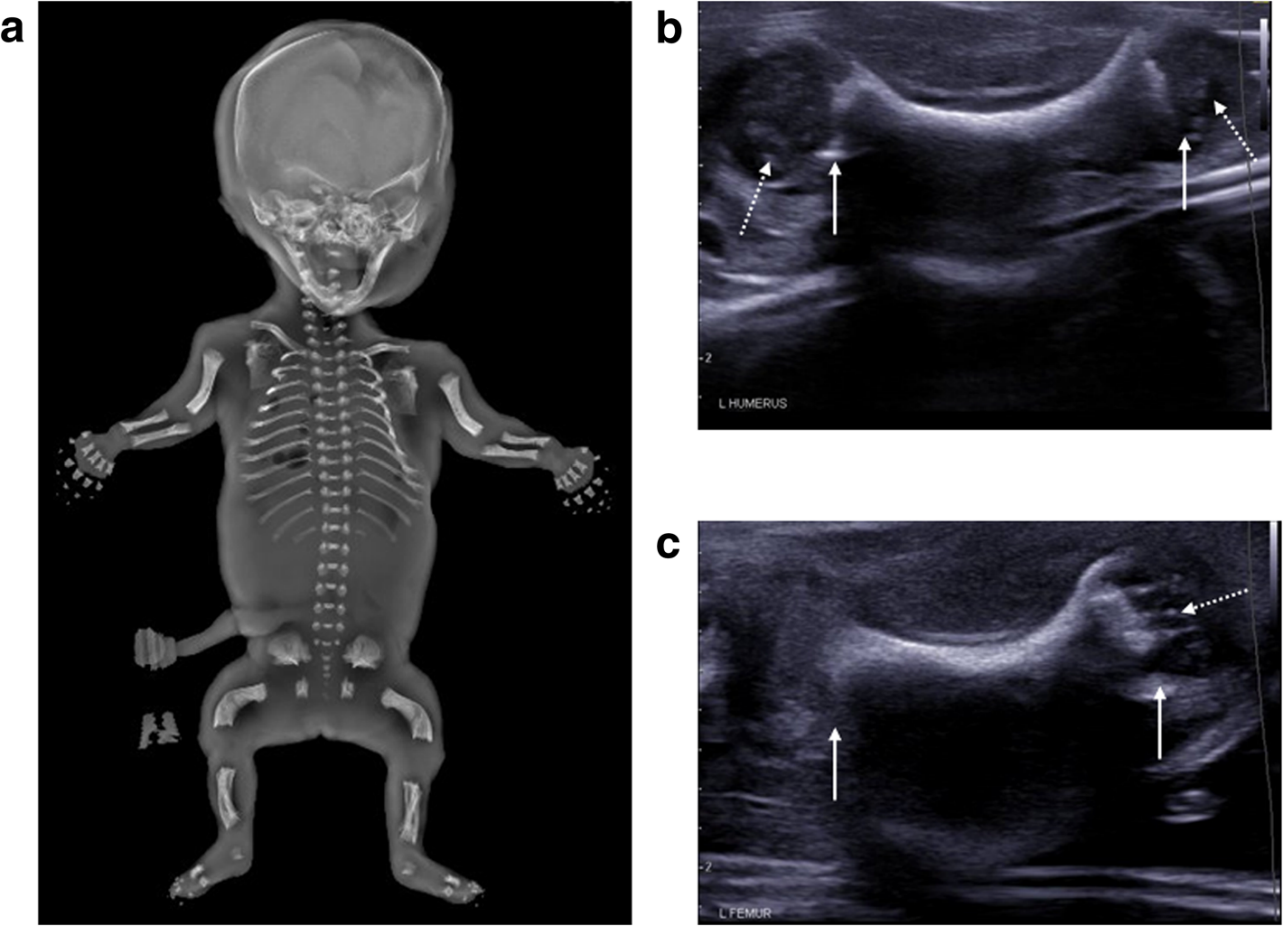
Thanatophoric dysplasia in a foetus at 22 weeks gestation, following termination of pregnancy. The frontal projection of the skeletal survey (a), acquired 2 days after delivery, demonstrates shortened long bones and curved femora with a trident appearance to the acetabulae. Post-mortem ultrasound images in sagittal plane of the left humerus (b) and left femur (c) reveal irregular metaphyses (solid arrows) and curved long bones. On ultrasound, it is also possible to visualise the unossified epiphyses (dotted arrows)
